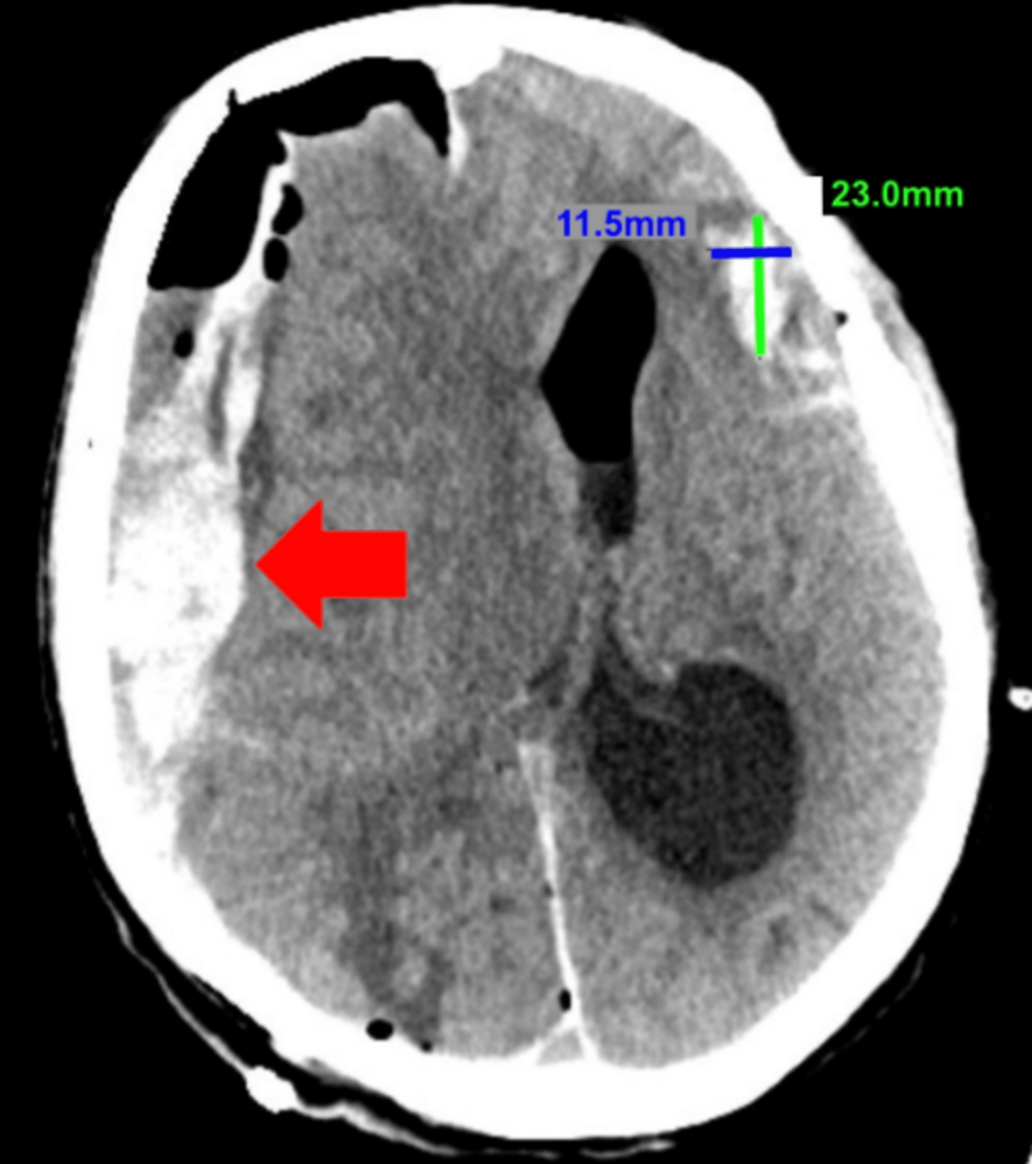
Non-contrast CT brain post-procedure depicting the partial resolution of intracranial air and a new right-sided SDH depicted by the arrow. There is also a small bleed on the left side, measuring 23.0 mm by 11.5 mm. SDH: Subdural hematoma
